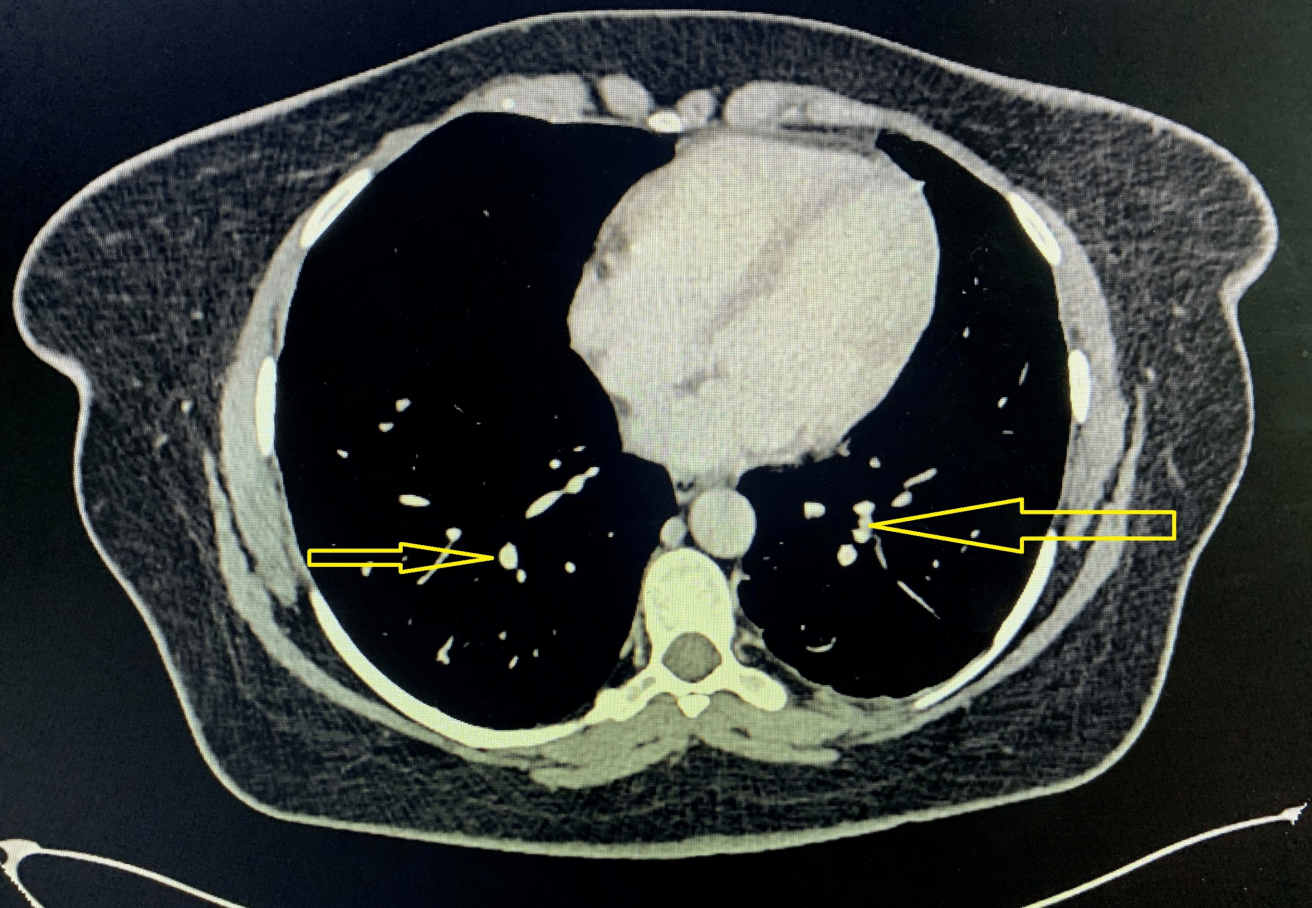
Chest CT scan showing multiple pulmonary nodules with surrounding ground-glass attenuation, suggestive of an invasive fungal infection
